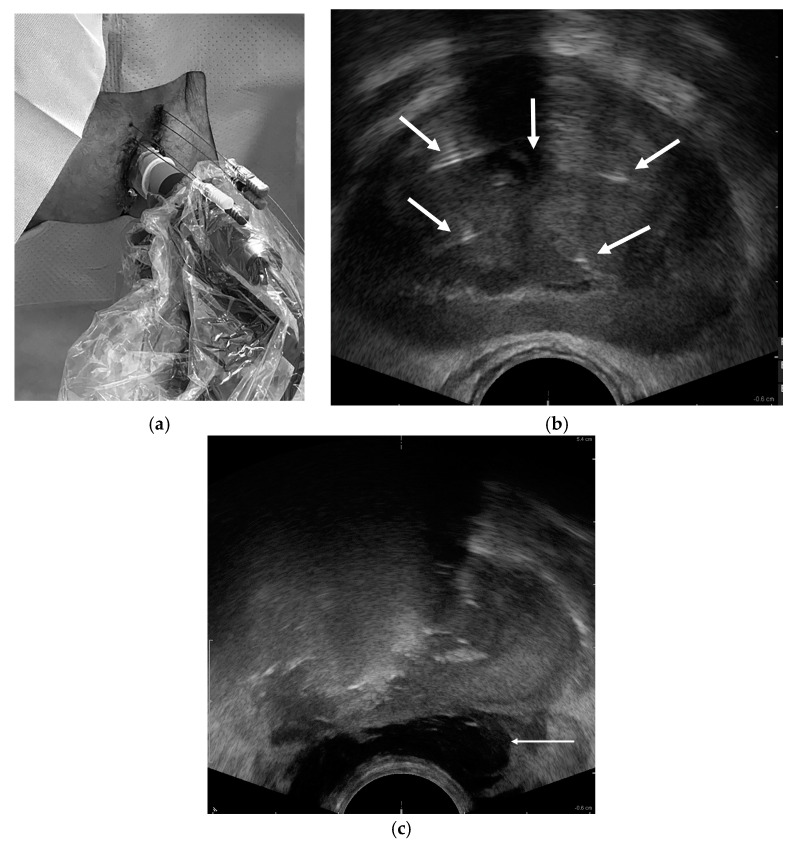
EchoLaser TPLA^TM^ (transperineal FLA) for BPH. (a) Focused placement of four 21 g needles using transrectal ultrasound guidance. The ultrasound probe is attached to a stabilizer (stepper) to enable stable guidance during needle placement. (b) Axial transrectal ultrasound image shows shadowing from Foley catheter centrally (middle arrow) and 4 periurethral needles for laser fiber positioning and ablation. (c) Right gland shadowing during active laser ablation and hydrodissection fluid injection in rectoprostatic space (arrow).
